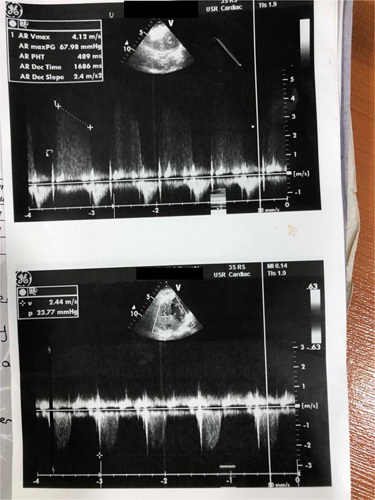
Echocardiogram of the patient. AR, aortic regurgitation; maxPG, maximum pressure gradient; PHT, pressure half-time.
